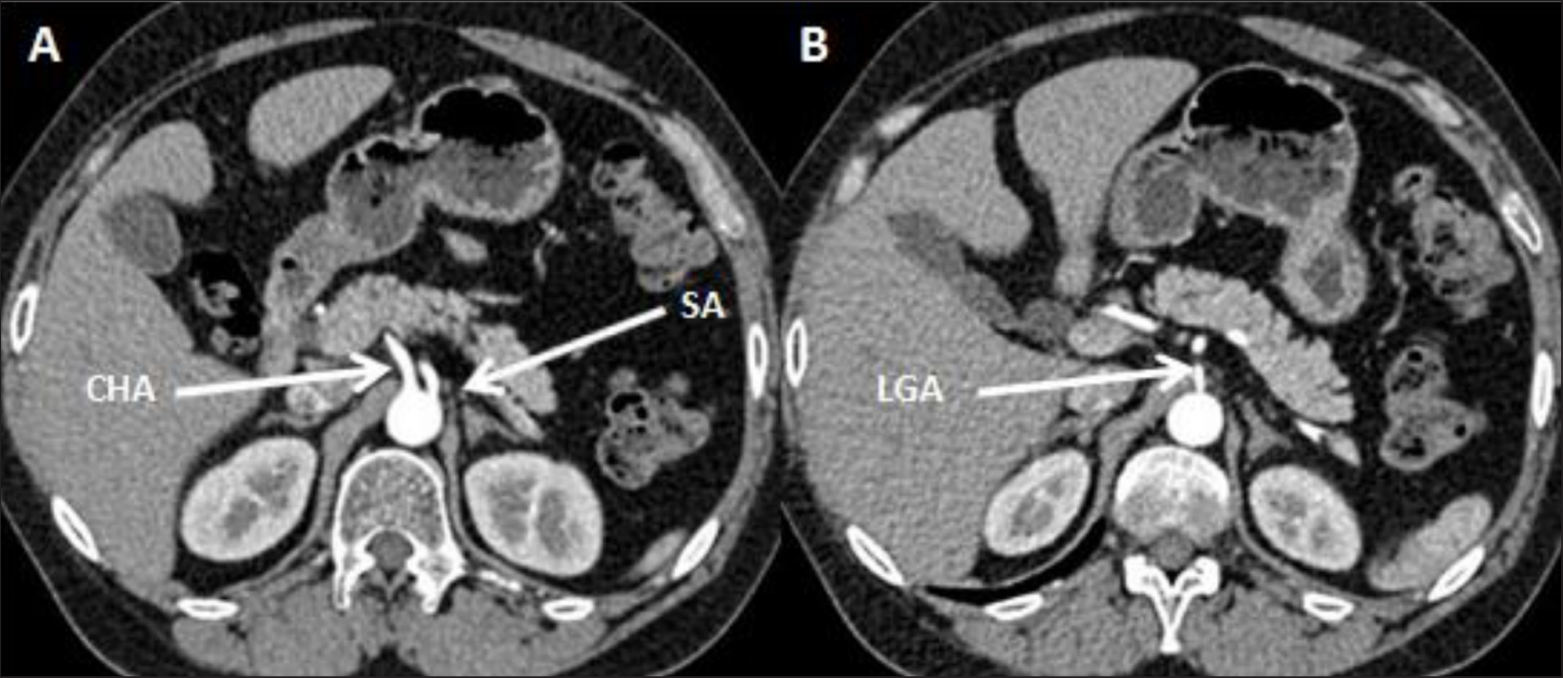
Abdominal CT scan in arterial phase demonstrate absence of the celiac trunk; the left gastric artery (LGA), splenic artery (SA), and common hepatic artery (CHA) arise directly and independently from the abdominal aorta.
